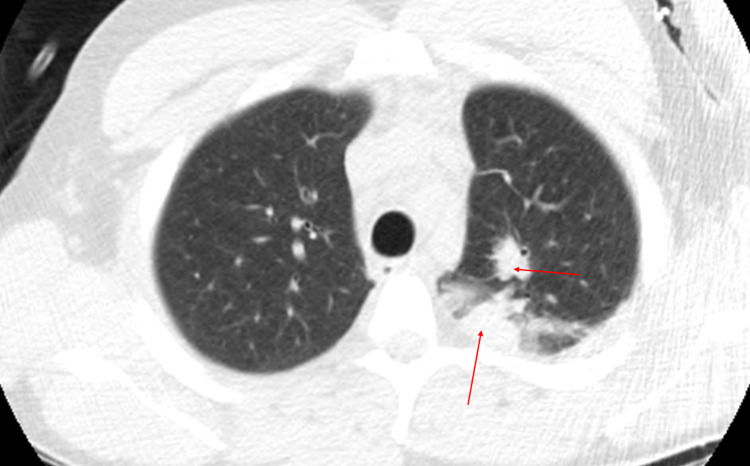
Thorax CT scan. Lung parenchyma hyperdensities in the upper left lobe (arrows).
